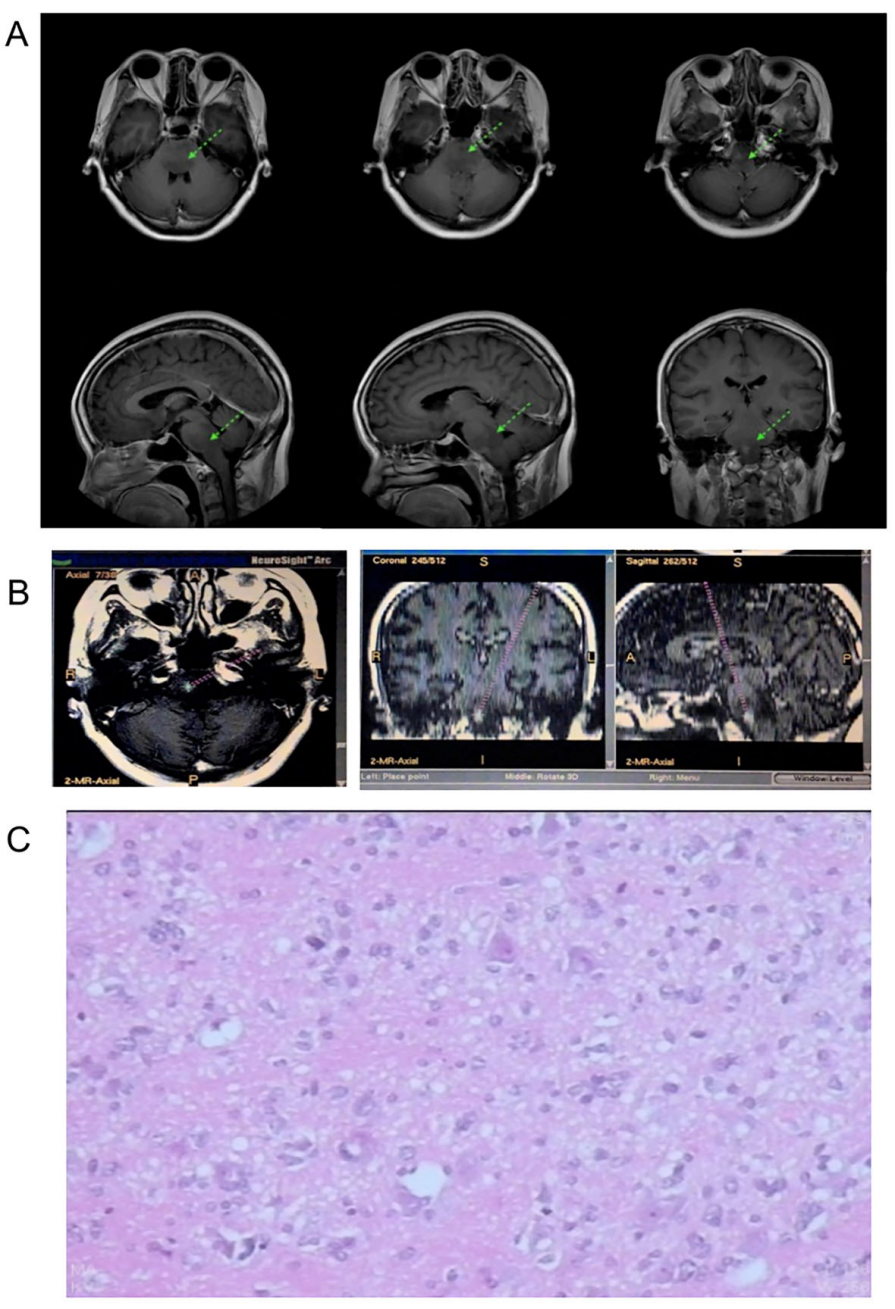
Case 3. A, T1-weighted contrast-enhanced magnetic resonance imaging showed pons, pons-medulla abnormally low signal, and no obvious enhancement. B, Surgical planning for micro-targets across the midline and pons-medulla junction. C, Pathological diagnosis of WHO Grade III gliomas.
